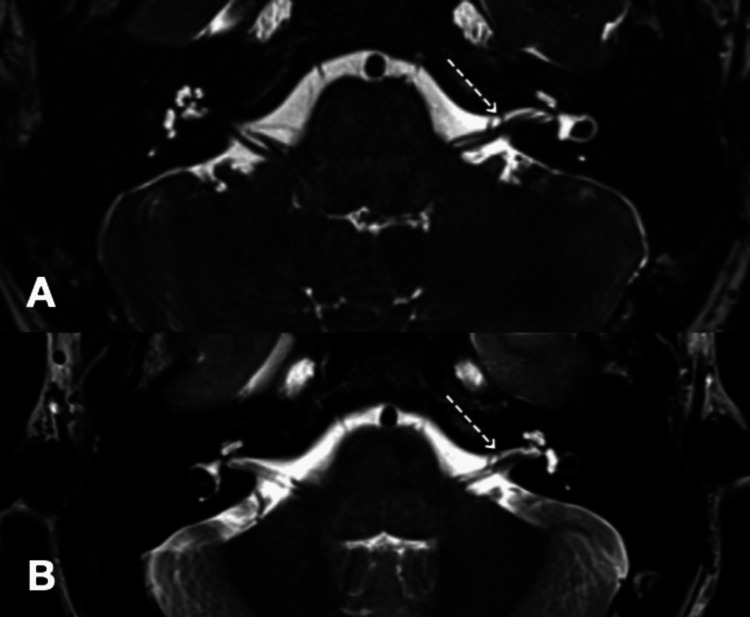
Magnetic resonance image analysis of Case 2 of constructive interference in steady state (CISS) sequence (A) and axial T2 (B) showing a vascular loop of the anterior inferior cerebellar artery (AICA) causing mild indentation over the left seventh and eighth cranial nerve complex (dotted arrow) at the entrance of the left internal auditory canal.
